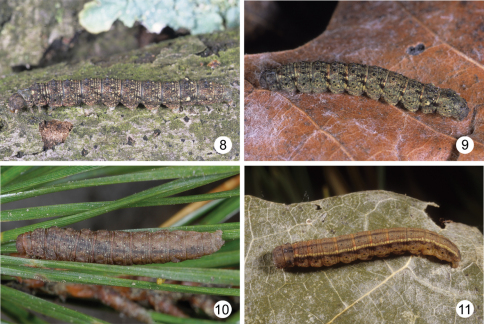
Last instar *Zanclognatha*. 8 *Zanclognatha dentata* sp. n.: NC: Haywood Co., Great Smoky Mountain National Park, Catalochee Campground, DLW Lot 2002E83, larva and photo 10 June 2002. 9 *Zanclognatha dentata* sp. n.: NJ: Atlantic Co., Egg Harbor Township, Abescon Creek, female (mother) 15 July 2002; photo: 24 August 2002, DLW Lot 2002G117 10 *Zanclognatha martha*. NY: Clinton Co., Clintonville, Dry Bridge Road, N44°28'14", W73°36'15", 660ft, larva 25 June, 2008, on *Pinus rigida*, DLW Lot 2008F200 11 *Zanclognatha protumnusalis*. CT: New London Co., Griswold, Hopeville Pond State Park, female 10 August, 1997, image November 1997, Fred Hohn lot number F263.
